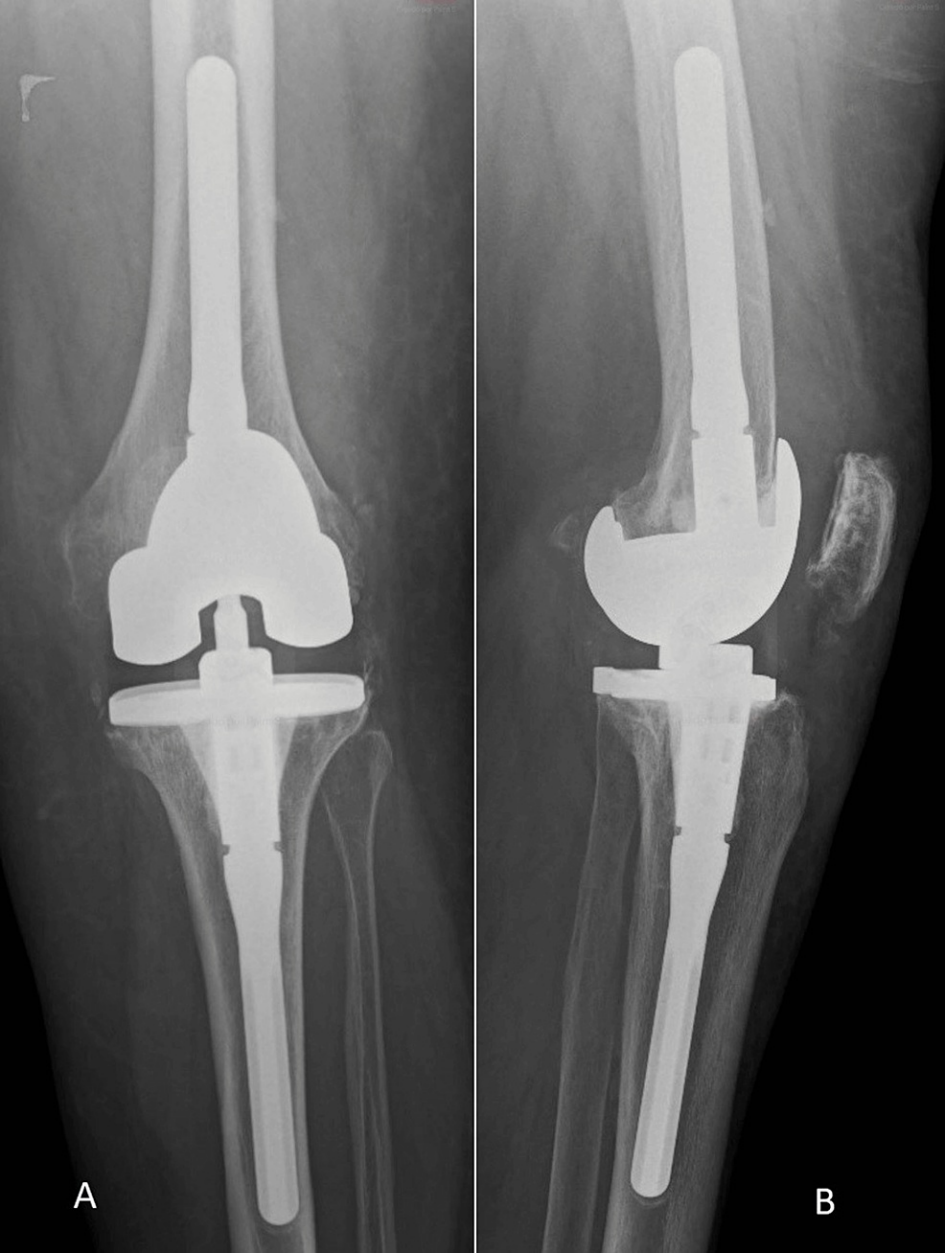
Anteroposterior (A) and lateral (B) radiographs of the revision total knee arthroplasty previous to the haematogenous infection.
